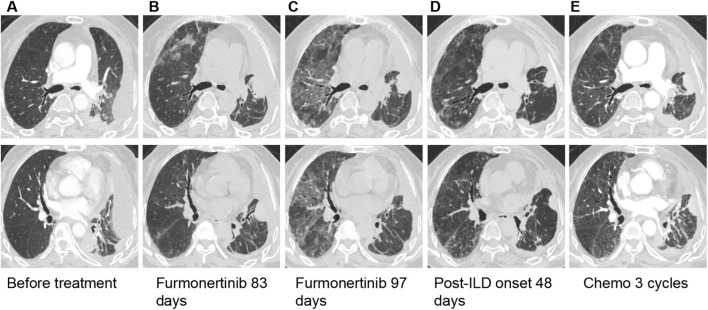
The chest CT of patient. (A) Chest CT showed the lung quality before furmonertinib (no evidence of interstitial lung disease). (B) Chest CT showed SD 83 days after furmonertinib therapy. (C) Chest CT showed pneumonia 97 days after furmonertinib therapy, the grid changes and ground glass hinting on lung injury. (D) The follow-up chest CT, performed 48 days after the onset of ILD, showed resolution of the drug-induced nonspecific interstitial pneumonia (NSIP) pattern. (E) Chest CT performed after three cycles of chemotherapy demonstrated no worsening of interstitial pneumonia and that the lung lesions remained radiologically stable.
